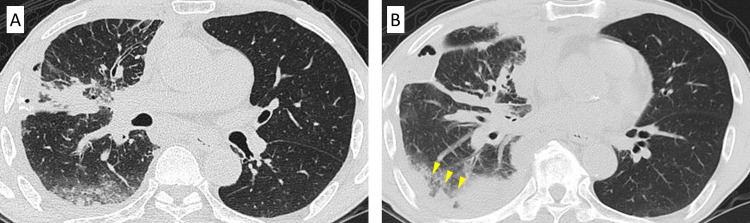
Chest computed tomography on day 7 after initiation of treatment with pembrolizumab Chest computed tomography on day 7 after initiation of treatment with pembrolizumab showed that pleural effusion worsened (yellow arrows).
